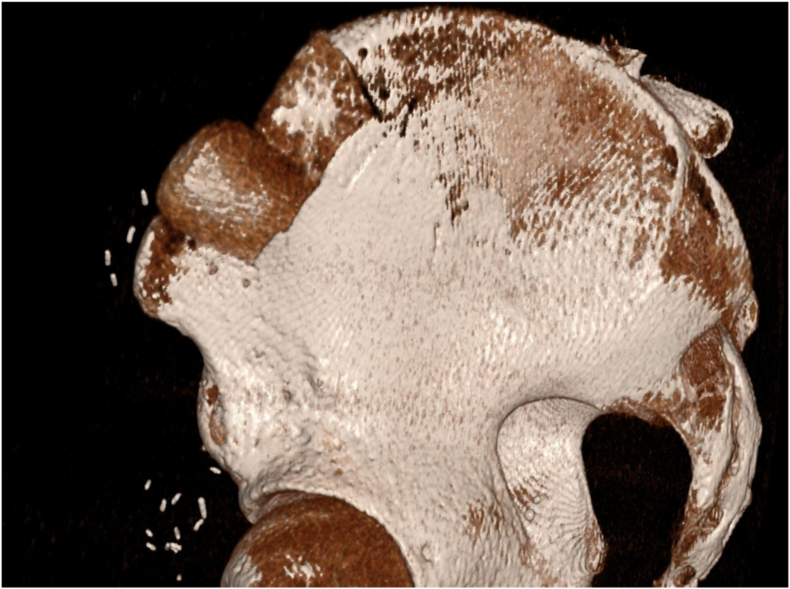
Early postoperative CT of an osteocutaneous SCIP donor site with bone allografts (Tutoplast®) in place. Additionally, the iliac wing fracture starting from the donor site can be seen.
